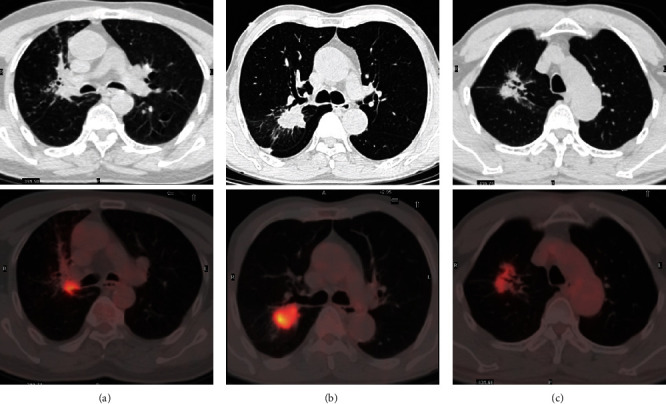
^18^F-FDG PET/CT findings of pulmonary lesions of PLC (a), atypical pulmonary metastasis of CRC (b), and atypical benign nodule (c). (a) A 68-year-old man with a history of rectal carcinoma. ^18^F-FDG PET/CT revealed a pulmonary lesion with air bronchogram (SUV_max_, 8.4; D_max_, 24 mm). The patient underwent pulmonary surgery. Pathological examination confirmed PLC. (b) A 64-year-old man with a history of rectal carcinoma. ^18^F-FDG PET/CT revealed a pulmonary lesion that was suspected to be malignant (SUV_max_, 9.93; D_max_, 32 mm). Pulmonary surgery confirmed pulmonary metastasis. (c) A 62-year-old man with a history of rectal carcinoma.^18^F-FDG PET/CT revealed a pulmonary lesion that was suspected to be malignant (SUV_max_, 3.84; D_max_, 14 mm), pleural indentation, and spiculation. Pulmonary surgery confirmed inflammatory disease.
